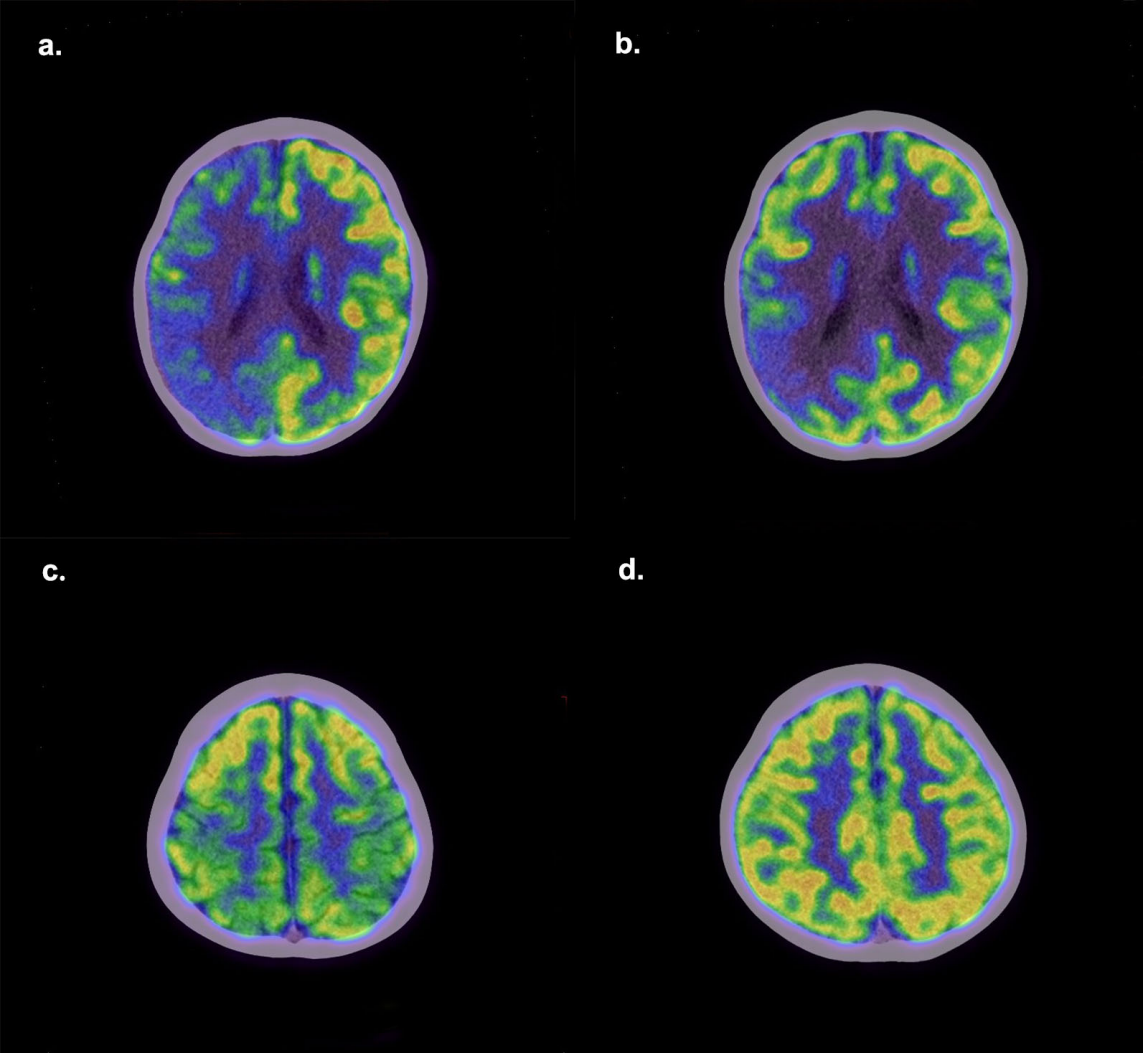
Representative PET images of patients with poor and good outcomes. Upper: A boy with AE had poor epilepsy control. His initial CASE score was 5, and three years after discharge, his CASE score remained 5. (a) The first 18F-FDG PET shows hypometabolism in the right cerebral cortex. (b) One year later, the second ^18^F-FDG PET shows there is still a seriously hypometabolic region in the right parietal lobe. Bottom: A boy had a 1-year history of gait instability and a 1-month history of severe language problems. Two years later, his language function has returned to normal, but gait in stability still exists. His CASE score has changed from 5 to 1. (c) The first ^18^F-FDG PET shows hypometabolism in bilateral parietal lobes. (d) Three months later, the second ^18^F-FDG PET shows the metabolism in the parietal lobe has recovered to normal.
